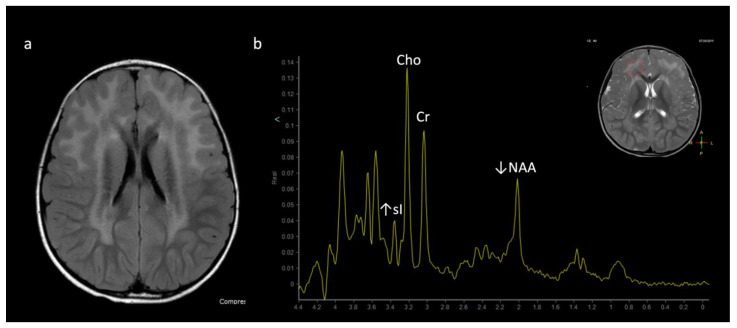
Alexander disease in a 3-year-old female with dysmorphic features and developmental delay. (a) Axial T2 FLAIR image demonstrates widespread hyperintense white matter signal involving the subcortical U fibers only in the frontal regions consistent with a leukodystrophy with an anteroposterior severity gradient. (b) SV-MRS of the right frontal white matter shows slightly elevated Lac, reduced NAA, and elevated scyllo-inositol at 3.36.
